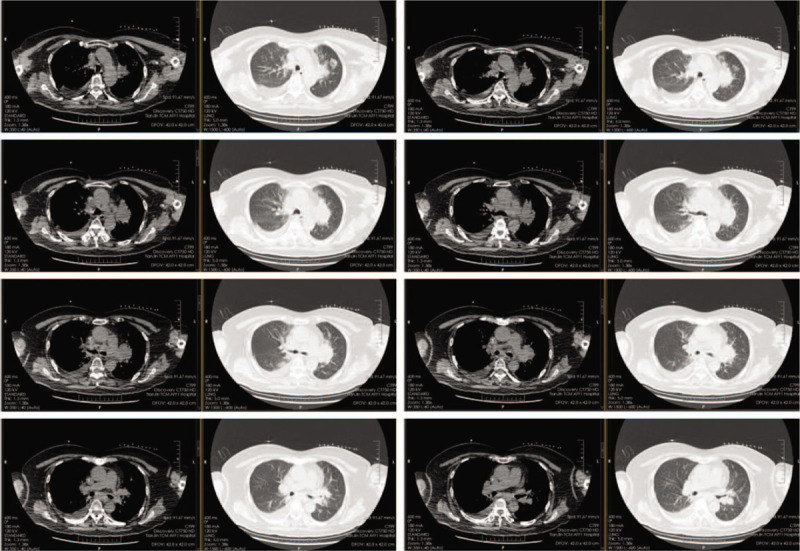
Chest computed tomography before iodine-125 radioactive seeds brachytherapy (May 24, 2018).
